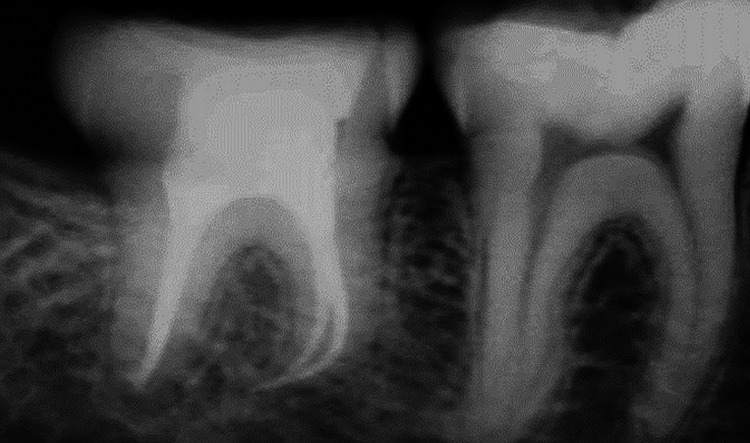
Radiographic evaluation of the 48 tooth after transplantation
